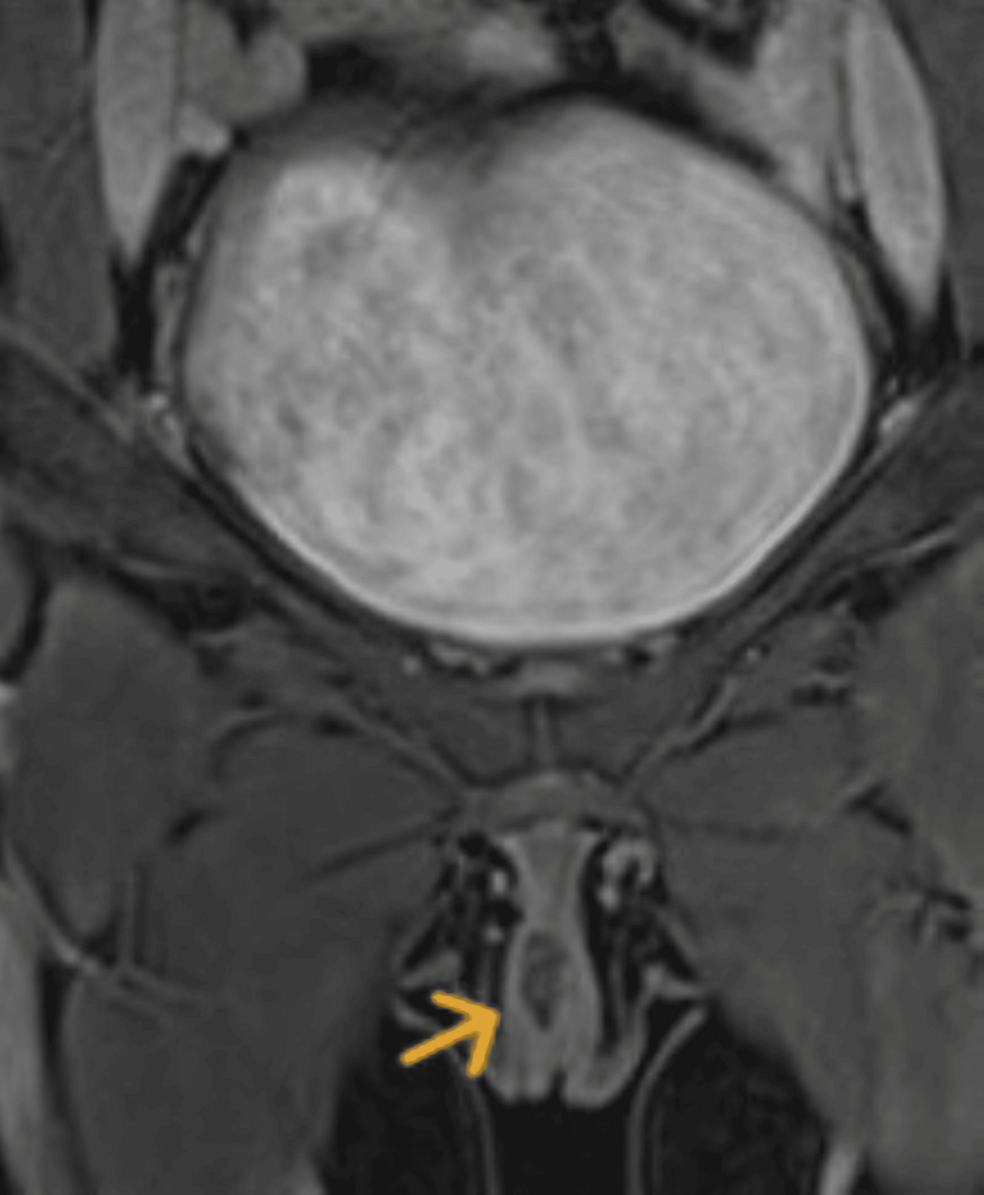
MRI showing a clitoral cyst.
